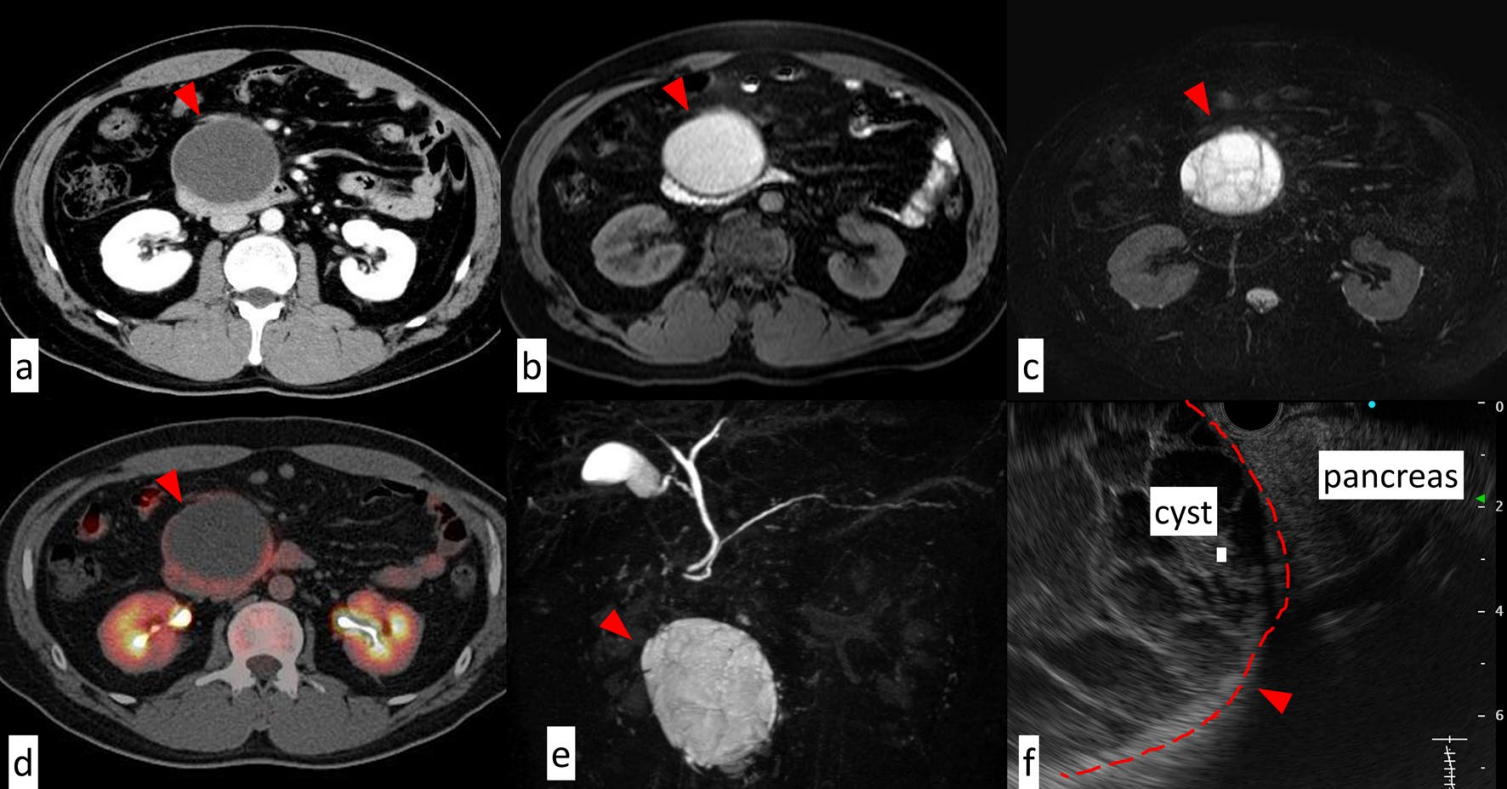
Images. Dynamic computed tomography (CT) showed the cyst, 10 cm in diameter, at the uncinate process of the pancreas without contrast enhancement (a). In magnetic resonance imaging (MRI), the cyst had homogeneous high signal intensity on T1-weighted images (b) and inhomogeneous signal intensity on T2-weighted images (c). No abnormal fluorodeoxyglucose (FDG) accumulation was observed on the FDG-positron emission tomography (PET) images (d). In MRCP, the cyst had no communication with pancreatic duct (e). Endoscopic ultrasonography (EUS) showed the cyst compressing pancreas, not arising from it (f)
